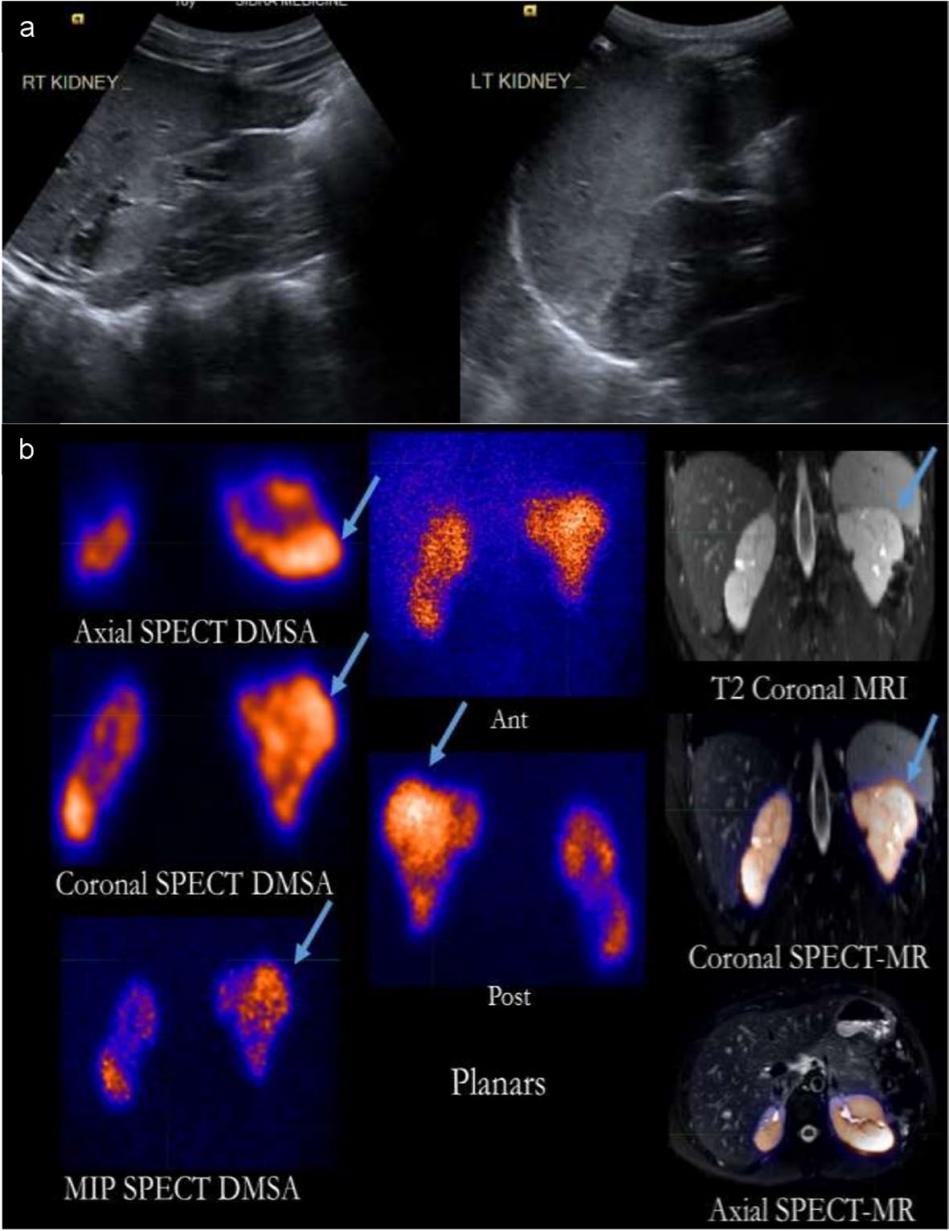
A US images of both kidneys demonstrate bilateral irregularity of the renal contours and multiple areas of renal scarring. B 16-year-old boy with CKD and bilateral abnormal kidneys on US. A large nodule is shown with a blue arrow on SPECT DMSA images and correlated on MRI of the abdomen. Increased background uptake is also noted on DMSA scans because of poor underlying renal function. Uptake in this nodular mass in the upper pole of the left kidney is diagnsotic of a FPT. Abbreviations: MR: Magnetic resenonace. MIP: Maximum intensity projection
